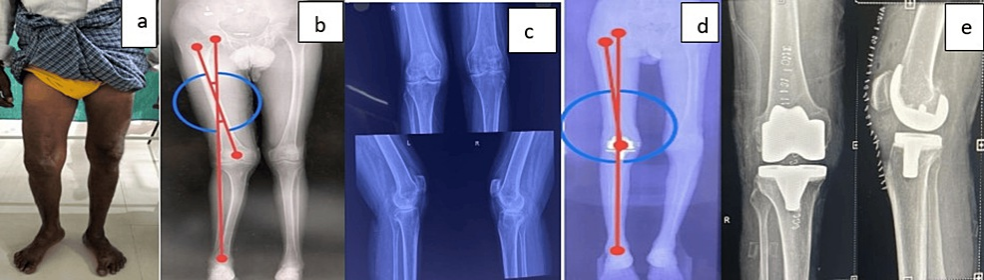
Case 2 (a) Preoperative clinical photograph. (b) Preoperative scanogram. (c) Preoperative radiograph. (d) Postoperative scanogram. (e) Postoperative radiograph.
